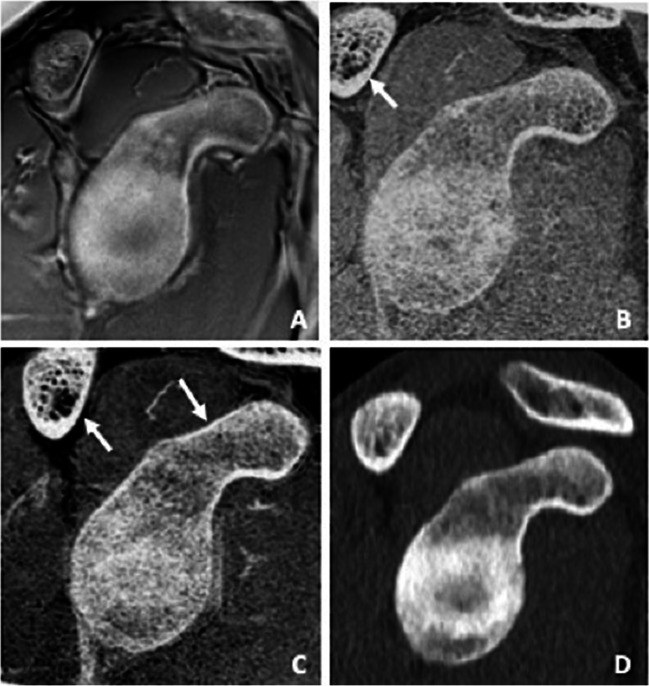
Intact glenoid of an 18-year-old patient with suspected traumatic shoulder injury, comparing sagittal images of an UTE, T1 GRE, and FRACTURE MR-sequence (A–C) to a conventional CT scan (D). Note the detailed bone trabecula in the gradient echo sequences T1 GRE and FRACTURE (B and C) as well as the good bone to soft tissue contrast of the UTE sequence (A) compared to CT (D). Further notice the thickening of the cortical bone in the T1 GRE and FRACTURE sequence compared to the UTE sequence (white arrows)
